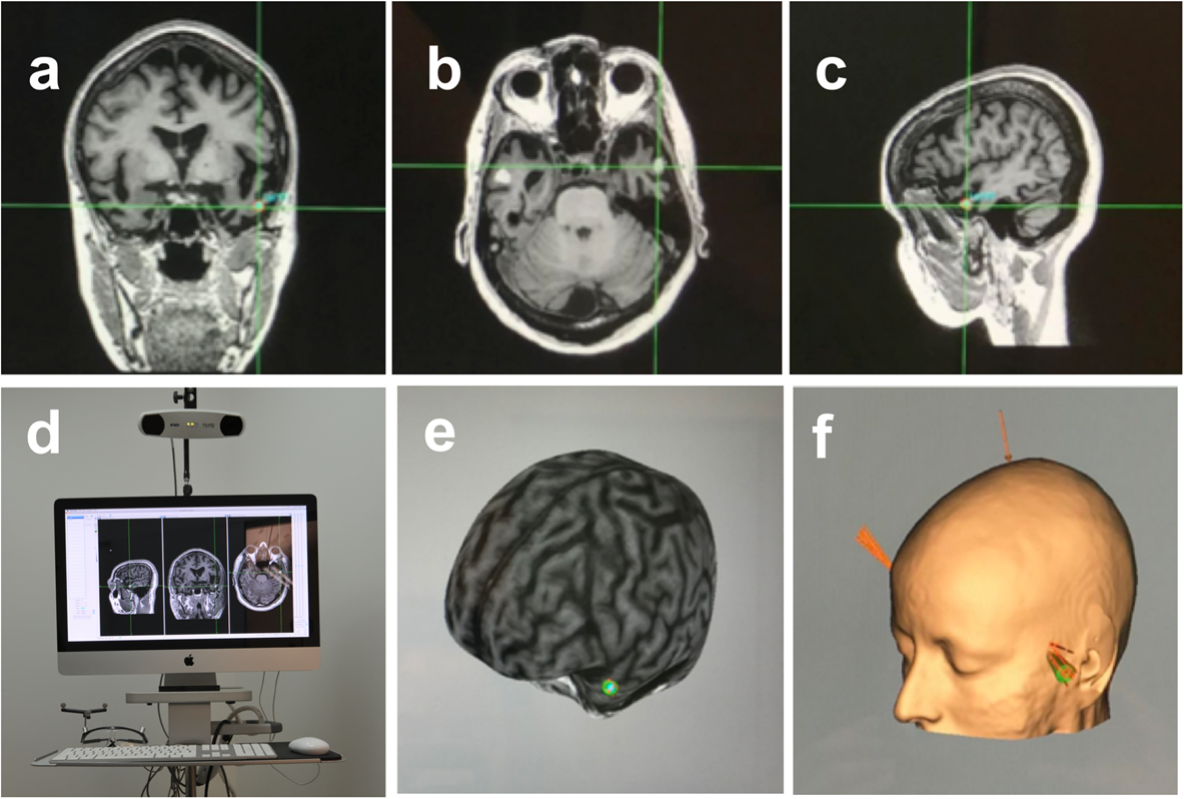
The three upper panels show, respectively, a coronal, b axial and c sagittal sections from magnetic resonance imaging (MRI) in a representative patient with semantic dementia (SD). The crosshair signals the location of the stimulation target in the left Anterior Temporal Lobe (ATL). d MRI-based frameless stereotactic navigation system device (Brainsight) employed for accurate targeting of the left ATL in SD patients. 3D brain curvilinear e cortical and f skin reconstructions from the MRI of a patient, provided by the neuronavigation system with a sphere indicating the left ATL target aimed by the stimulation
